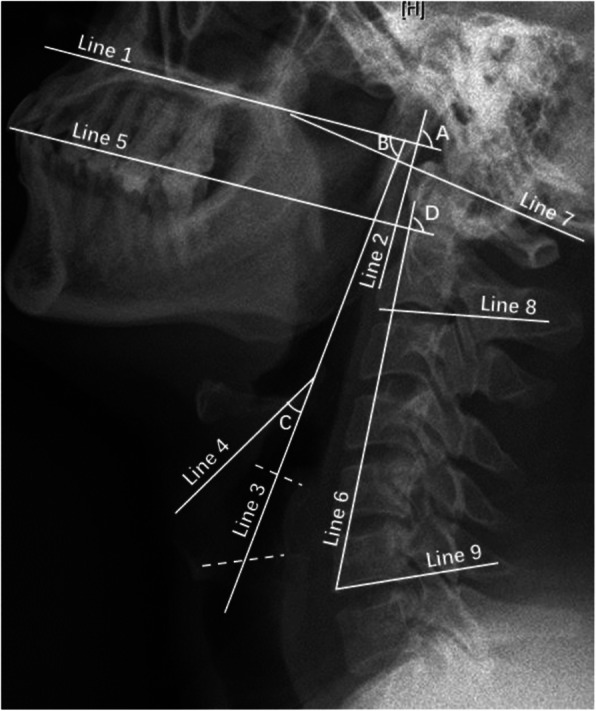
Angle indicators on the lateral cervical X-ray in the neutral position. Angle A: angle between Line 1 and Line 2; Angle B: angle between Line 1 and Line 3; Angle C: angle between Line 3 and Line 4; Angle D: angle between Line 5 and Line 6; Angle E: angle between Line 7 and Line 8; Angle F: angle between Line 8 and Line 9. (Line 1: a line parallel to hard palate; Line 2: a line passing through the anterior point of the bodies of atlas and axis; Line 3: a line passing through the airway midpoint crossing the cricoid cartilage; Line 4: a line parallel to epiglottis; Line 5: a line along the occlusal surface of maxillary teeth; Line 6: a line passing through anterior-inferior border of the sixth cervical vertebra and the most anterior aspect of the first cervical vertebra; Line 7: a line passing through the posterior-superior point of hard palate and the lowest point of the occipital bone; Line 8: a line passing through the anterior-inferior point and the posterior-inferior point of the second cervical vertebral body; Line 9: a line passing through the anterior-inferior point and the posterior-inferior point of the sixth vertebral body)
